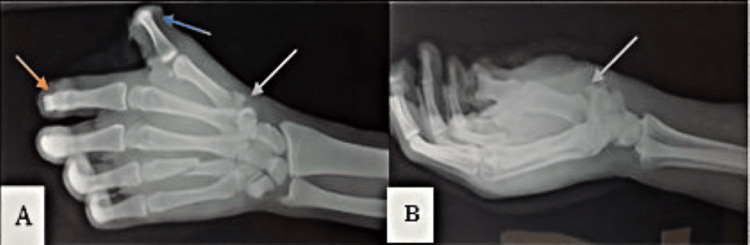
Standard radiographs of the hand. (A) Anteroposterior view and (B) lateral view, demonstrating the absence of the distal phalanges of the thumb (blue arrow) and second digit (orange arrow), with associated soft tissue loss. A midshaft fracture of the fourth metacarpal and a fracture at the base of the first metacarpal (gray arrow) are also noted.
